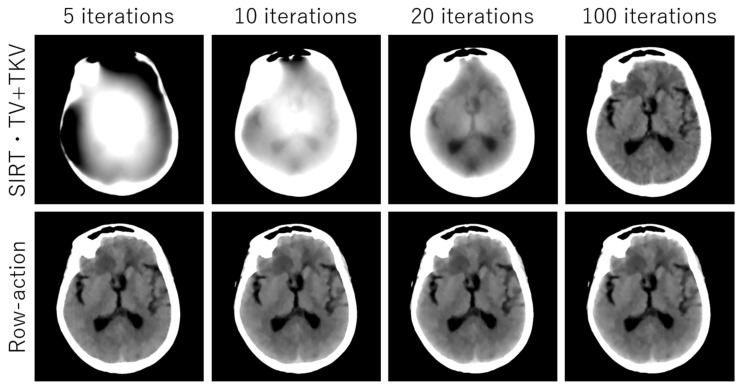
The reconstructed images of low-dose CT (256 projection data and the number of photon counts $3\times 10^{6}$). SIRT nonlocal TV+TKV and row-action accelerated nonlocal TV+TKV (our proposed method) were compared. All images are displayed with the same window of [7.82, 62.30] HU.
